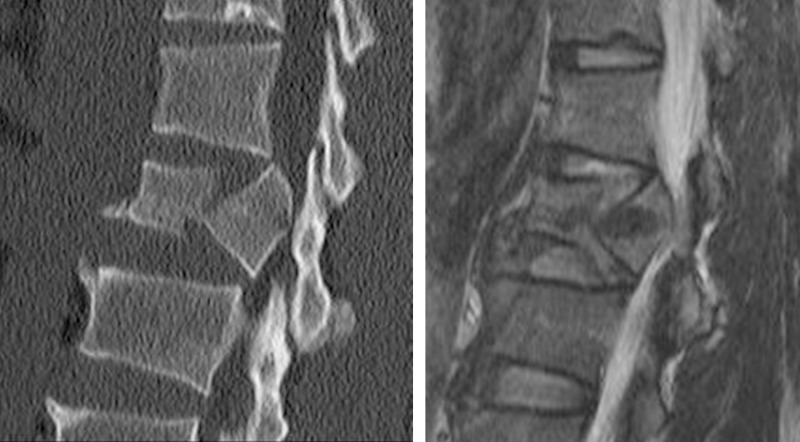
34-year-old man with an A3.1 fracture at level L2. T2WI magnetic resonance image shows that the upper intervertebral disc is injured at a Grade 3 level and that the lower disc is a Grade 1 injury.
